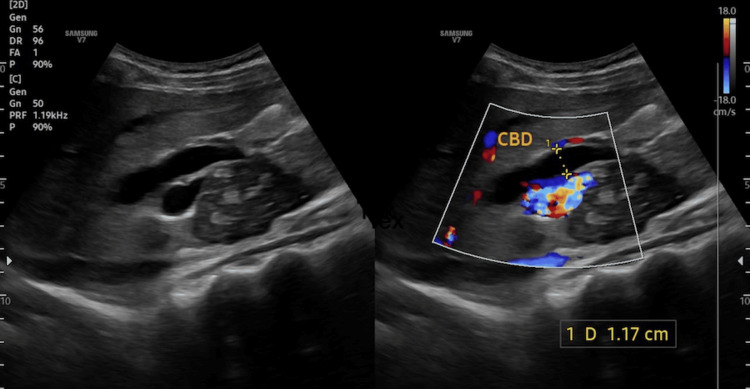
Transabdominal ultrasonography of the hepatobiliary system USG demonstrates dilatation of the common bile duct (CBD), measuring approximately 1.17 cm in diameter. The duct appears prominently dilated with evidence of upstream biliary dilatation. Color Doppler imaging shows no significant internal vascularity within the duct, supporting a non-vascular obstructive etiology.
